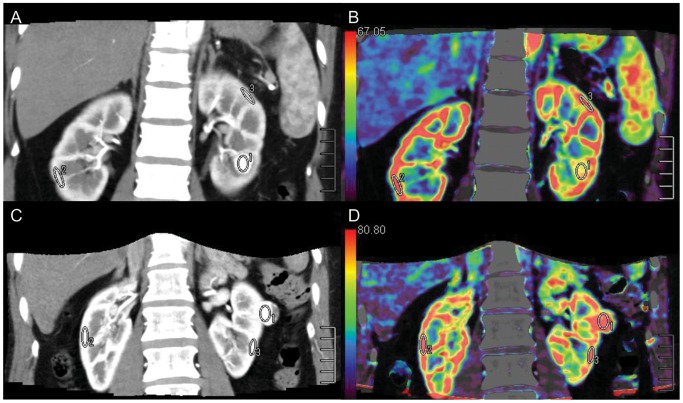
ROIs (ROI 1, ROI 2, and ROI 3) were placed in the tumor and in healthy ipsi- and contralateral renal cortex. ROI 1 was the ROI of tumor tissue. AML with minimal fat (A) (mean CT value = 255.4 HU) with a sufficient blood supply had an abnormal enhancement pattern that was similar to clear cell RCC (C) (mean CT value = 251.4 HU), while Equiv BV was much lower in AML (B) (mean Equiv BV = 57.7) than in clear cell RCC (D) (mean Equiv BV = 85.2).
